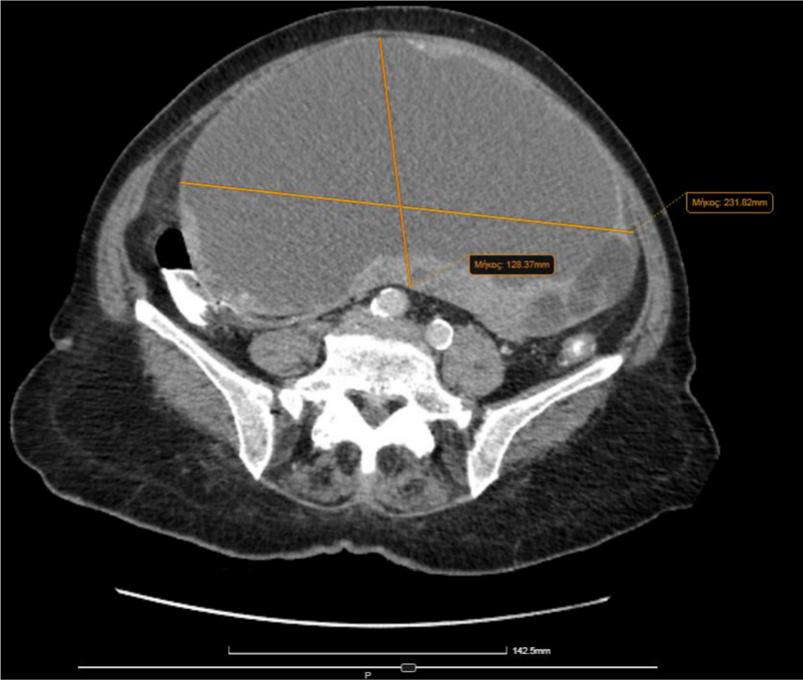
Computed tomography (CT) of the mass.
